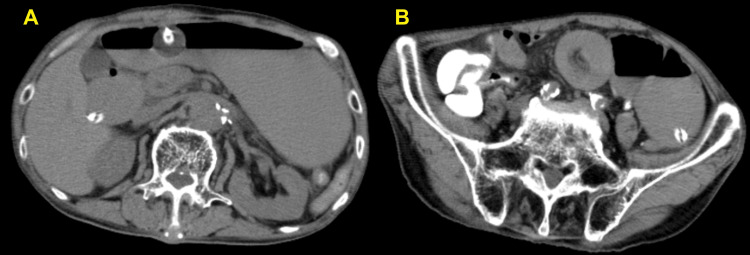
Abdominal CT scan at the day after PEG-J implantation (A) Worsening of ileus and (B) a new jejunal intussusception further anorectally from the catheter tip. CT: computed tomography; PEG-J: percutaneous endoscopic gastrojejunostomy
